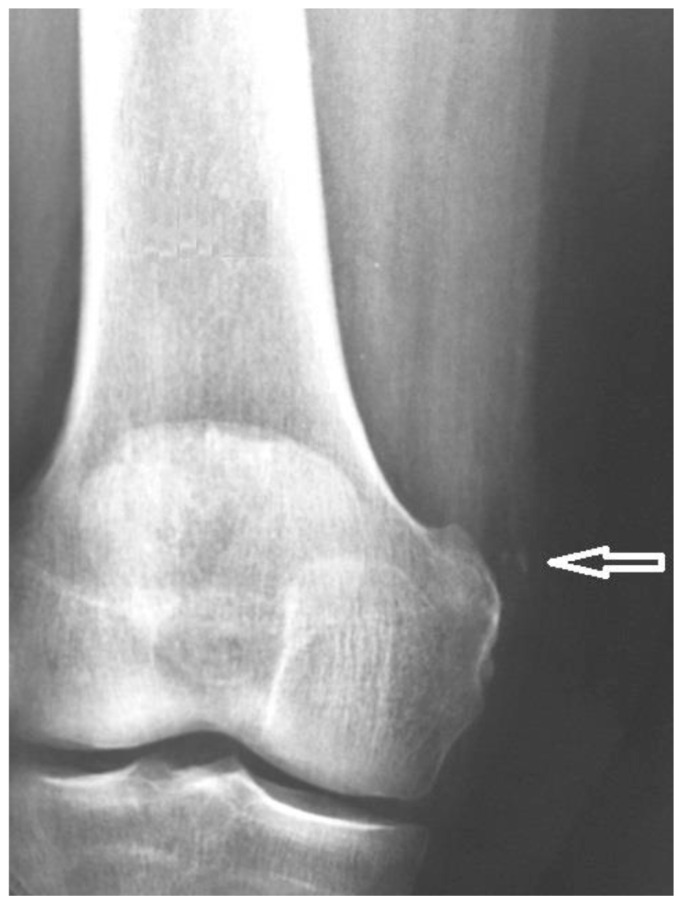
Anteroposterior radiograph at 1-month post-UGPL. Only small residual fragments of the calcification are visible (arrow). The patient was asymptomatic (EVA 0).
